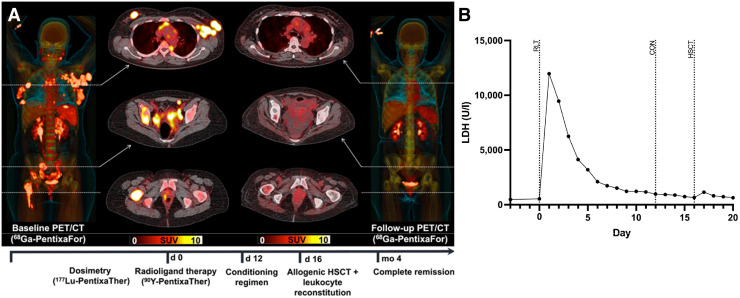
Patient with relapsed T-cell lymphoma treated with CXCR4-directed RLT and achieving complete remission. (A) Maximum-intensity projection and transaxial ^68^Ga-pentixafor PET/CT at baseline showed extensive disease in skeleton and lymph nodes. Four months after treatment, CR was noted on follow-up ^68^Ga-pentixafor PET/CT. (B) Lactate dehydrogenase as surrogate marker of antilymphoma efficacy peaked directly after RLT and then rapidly declined till conditioning regimen and HSCT, thereby suggesting direct antilymphoma effect caused by CXCR4 RLT. CON = conditioning regimen; LDH = lactate dehydrogenase. (Modified from (7).)
